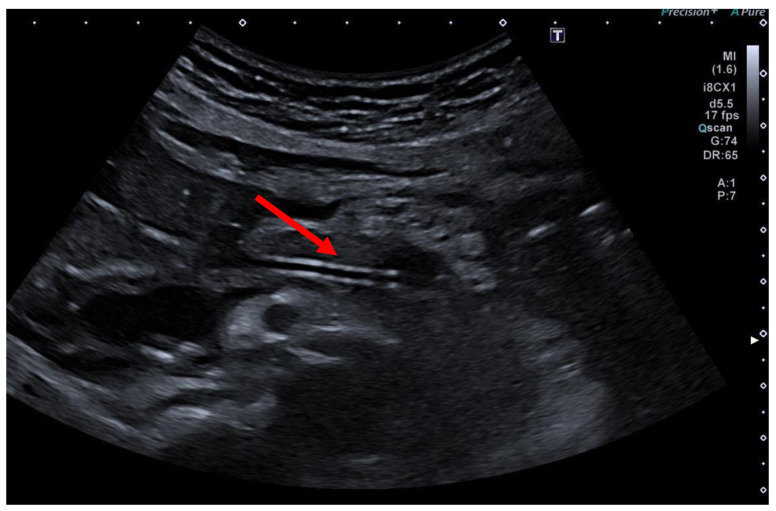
Postinterventional ultrasound control after EUS-PD revealing a decompressed pancreatic duct with a transgastric plastic stent in place (red arrow), selected from the clinical picture library of the reporting Dept. of Gastroenterology, Hepatology and General Internal Medicine.
